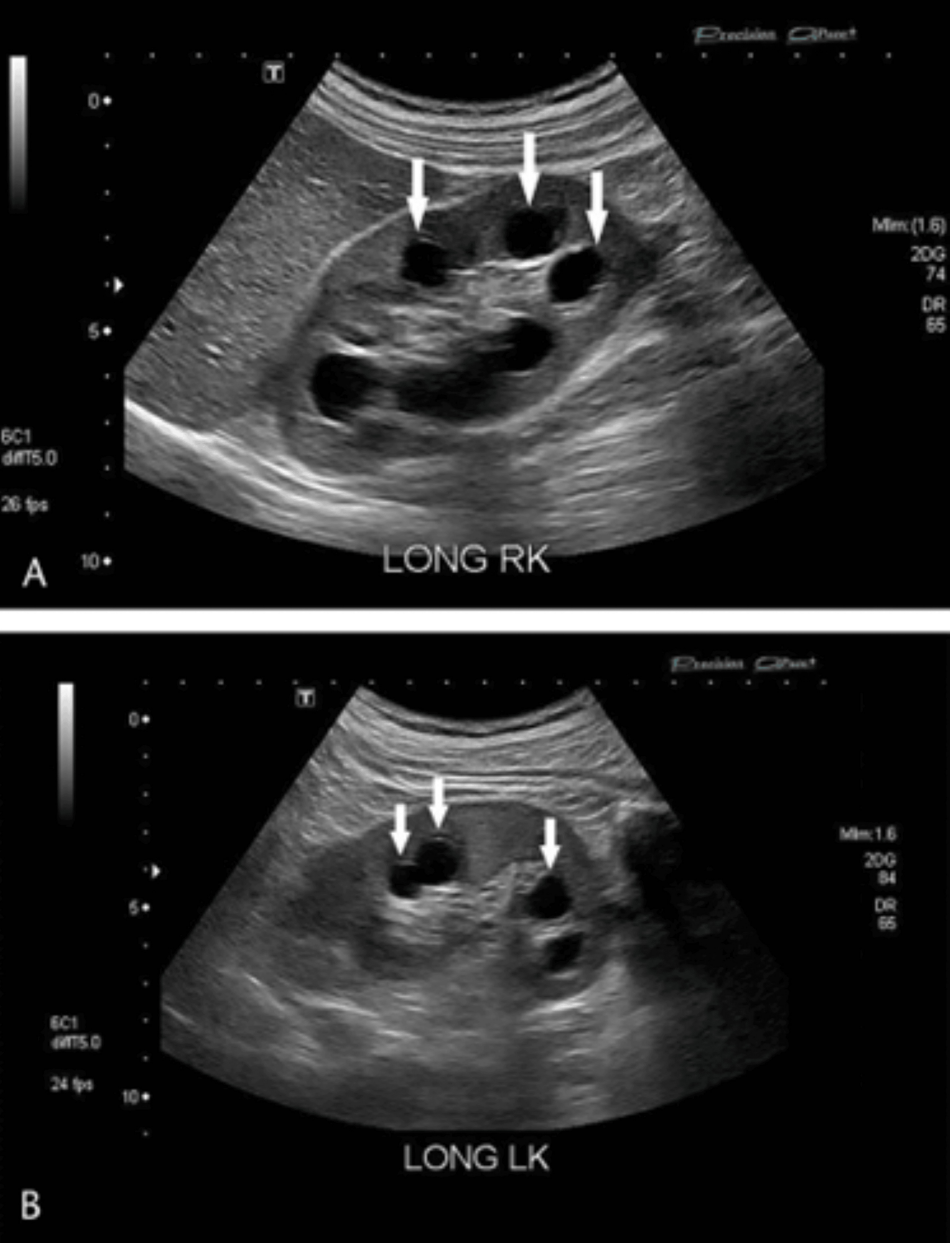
Longitudinal kidney ultrasound of a patient with medullary cystic kidney disease (MCKD). This image depicts multiple medullary cysts in the right (A) and left kidney (B). (Reproduced from Whang et al., with permission from Wolters Kluwer Health, Inc.) [2].
